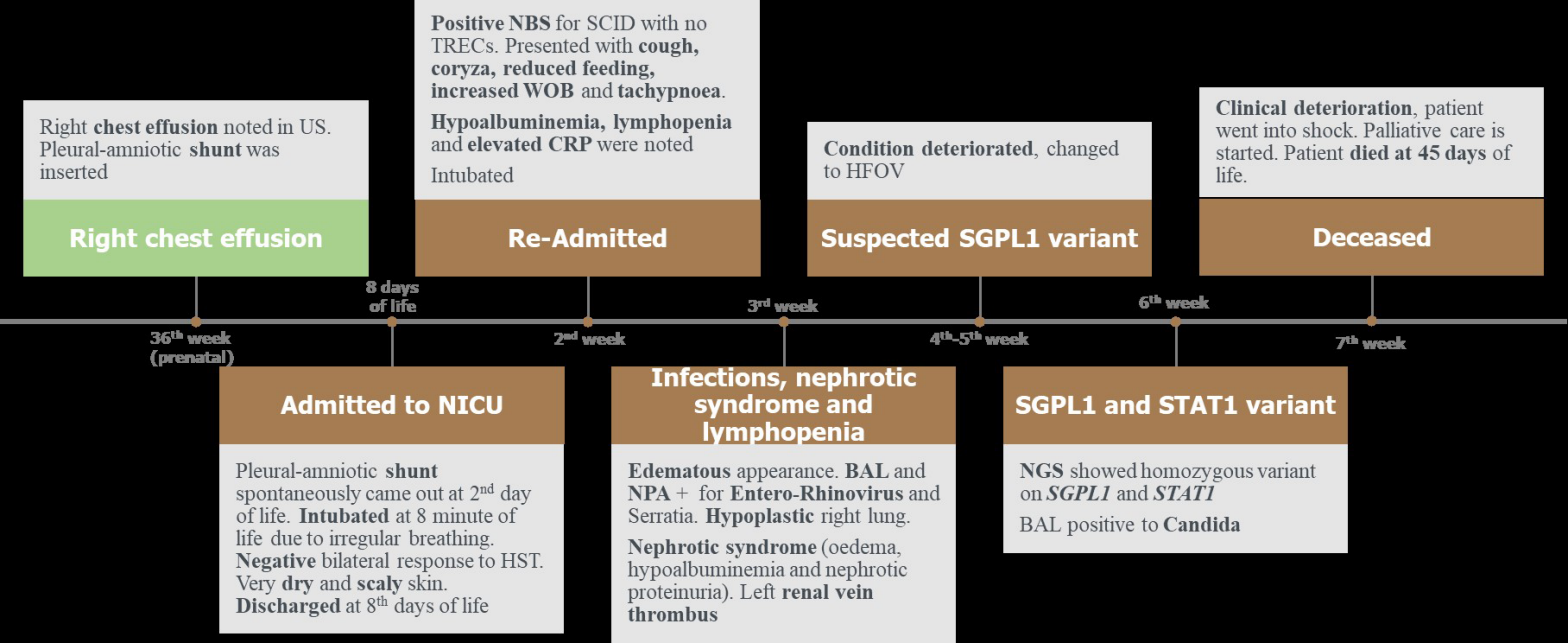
Timeline of the clinical presentation of the patient. BAL, bronchoalveolar lavage. CRP, C-reactive protein. HST, hearing screening test. HFOV, high frequency oscillatory ventilation. NBS, newborn screening. NPA, nasopharyngeal aspiration. NICU, neonatal intensive care unit. NGS, next generation sequencing. SCID, severe combined immunodeficiency. *STAT1*, signal transducer and activator of transcription. *SGPL1*, sphingosine 1-phosphate lyase. US, ultrasound. WOB, work of breathing.
